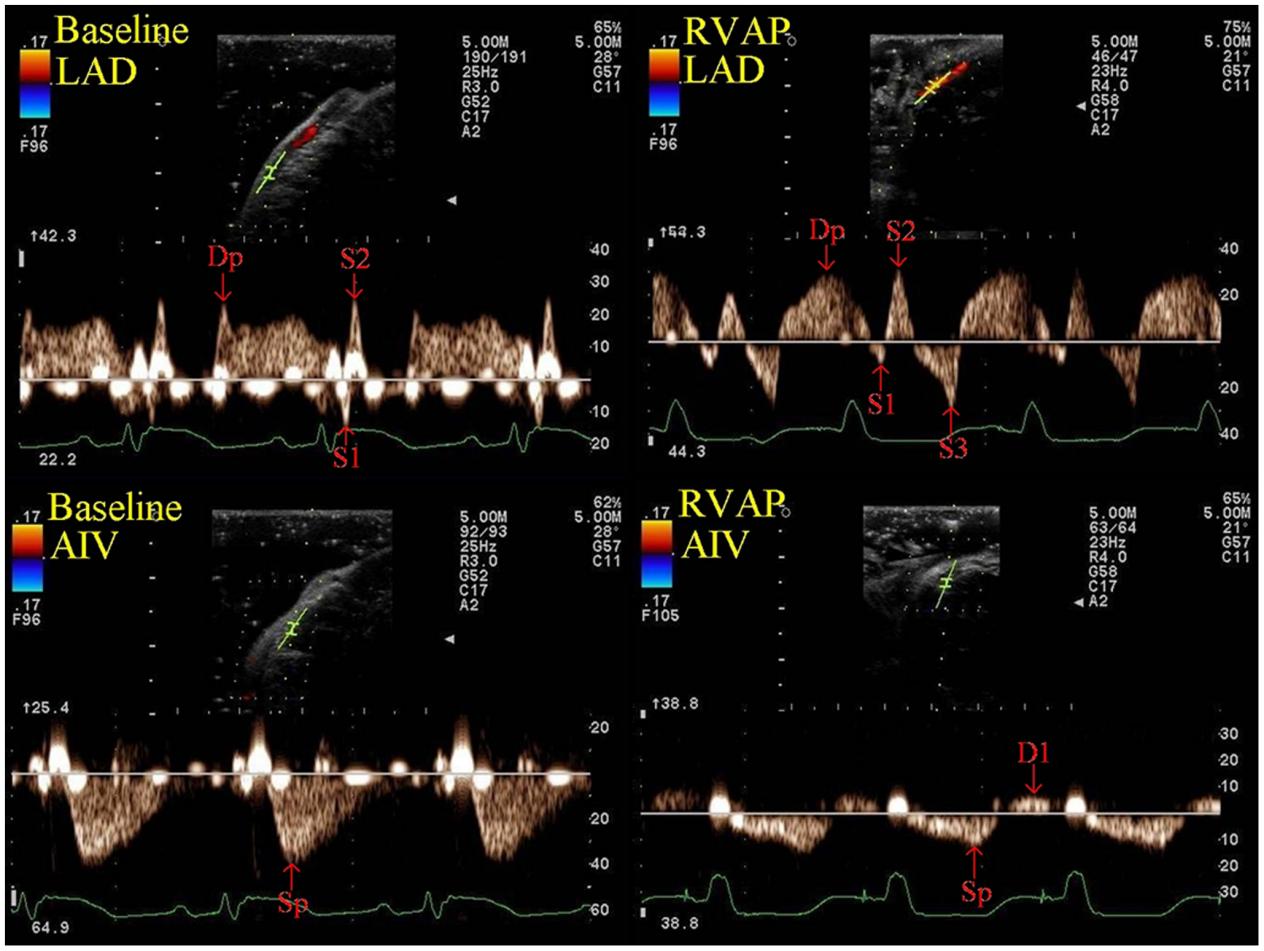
The spectral Doppler waveforms of the middle segment LAD and the AIV at both states in one open chest beagle. The spectral Doppler waveforms of the LAD at baseline condition had a principal positive wave (Dp) during diastole, and heeled by a momentary negative wave (S1) and a positive wave (S2) during early systole, and a transitory cessation flow appeared during mid to late systole (upper row left panel). During RVAP, a negative wave (S3) appeared at late systole that did not exist in the LAD at baseline condition (upper row right panel). The spectral Doppler waveforms of AIV only had a principal positive wave (Sp) at baseline condition, and the Sp appeared at the middle systole and lasting almost the whole diastole, and a momentary cessation flow appeared at the end of diastole (lower row left panel). During RVAP, the Sp of the AIV appeared at the early systole and heeled by a diastolic negative wave (D1) (lower row right panel). Baseline = baseline condition; RVAP = right ventricular apical pacing; LAD = left anterior descending coronary artery; AIV = anterior interventricular vein.
